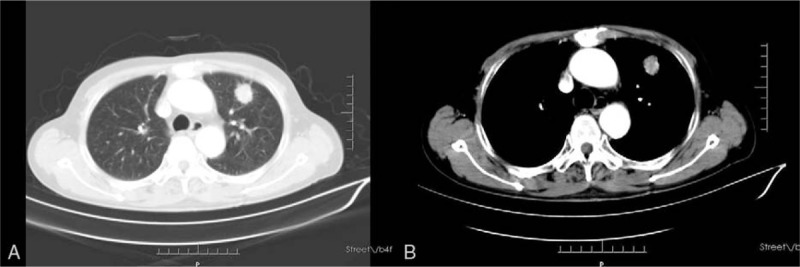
A, B. Chest and upper abdominal computed tomography (CT) showed a solitary nodule, measuring 2.3 cm in diameter, in the left superior lung lobe.
